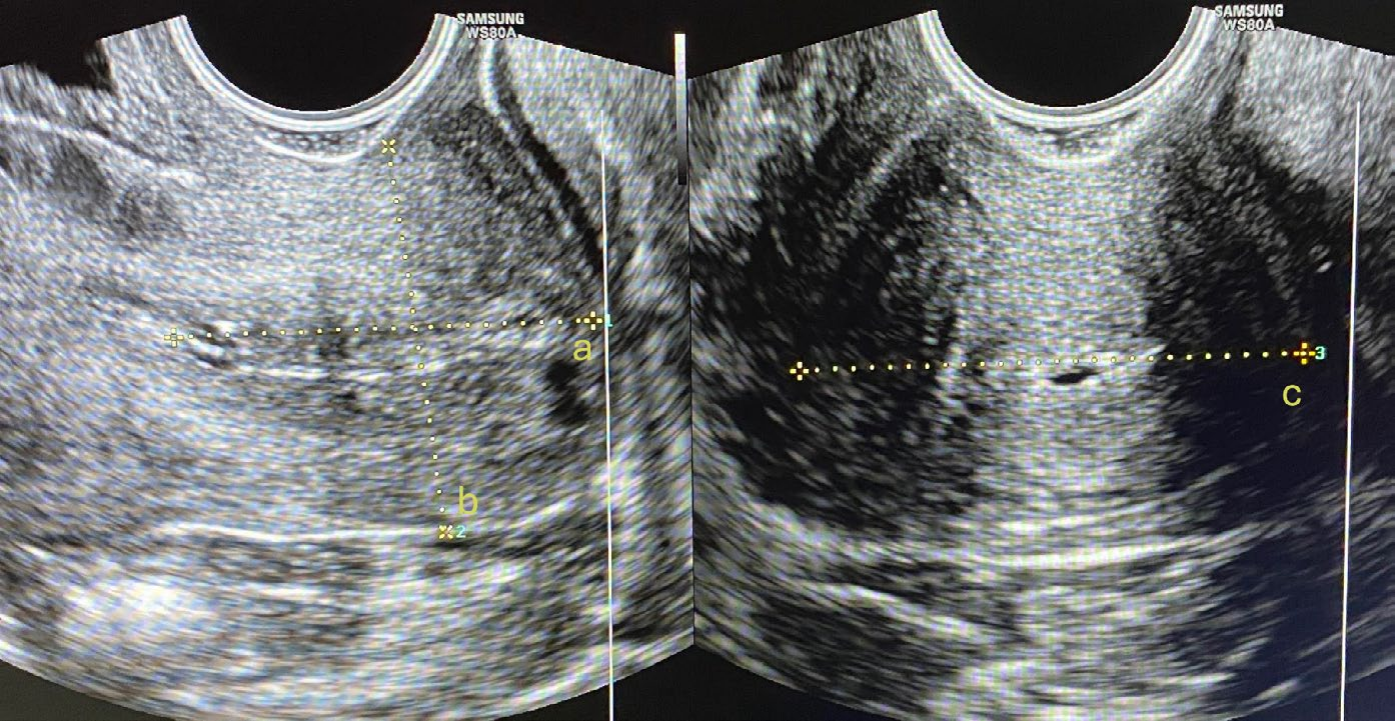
Cervical measurements using 2D ultrasound. (a) Cervical length and (b) anteroposterior (AP) diameter measured in the sagittal plane, and (c) transverse (T) diameter measured in the transverse plane.
